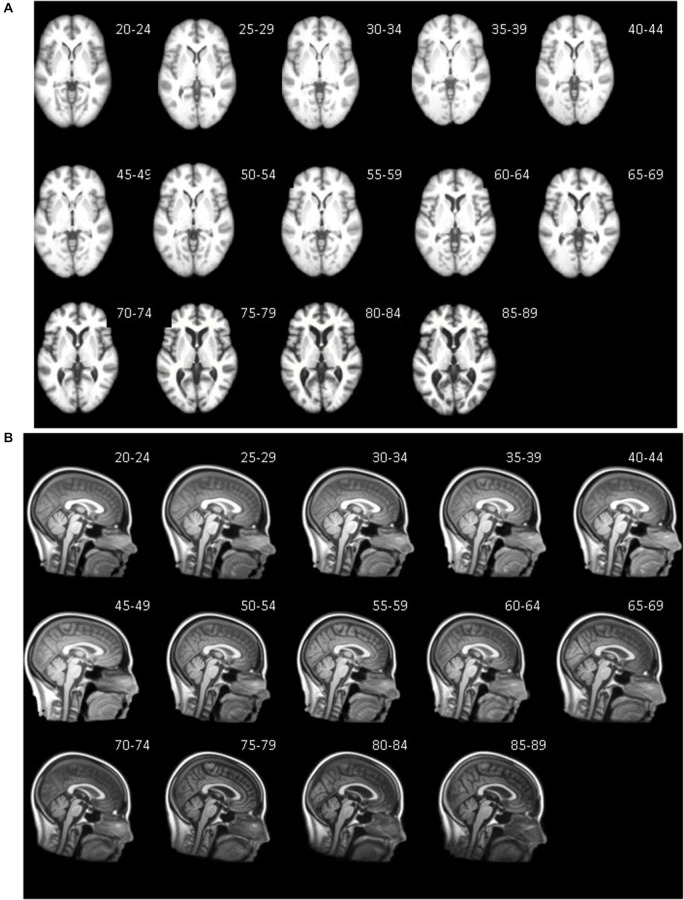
Age-specific templates showing axial slice at AC-PC commissure (A) of brain extracted average template and mid-sagittal slice (B) of whole head average template. T1W MRI volumes across ages of study.
